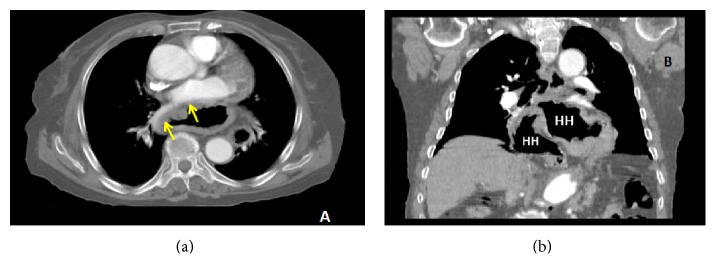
Axial and coronal images of the hiatal hernia on thoracoabdominal CT scan. (a) Compression to left atrium and right pulmonary vein by hiatal hernia on axial plane (yellow arrow); (b) herniation of majority of stomach into thorax cavity on coronal plane; HH: hiatal hernia.
